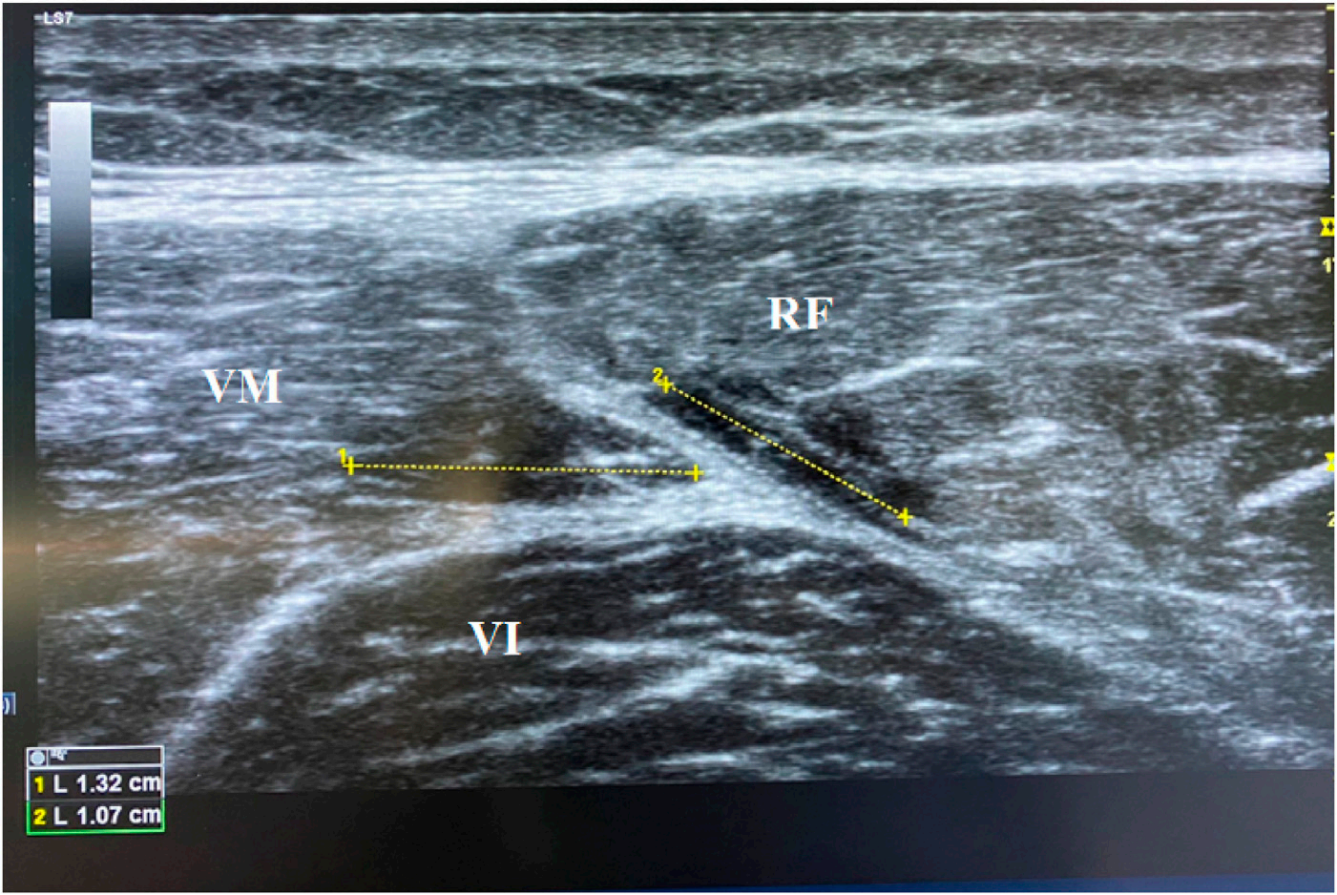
Ultrasonography of the rectus femoris 1 week post-injury.
